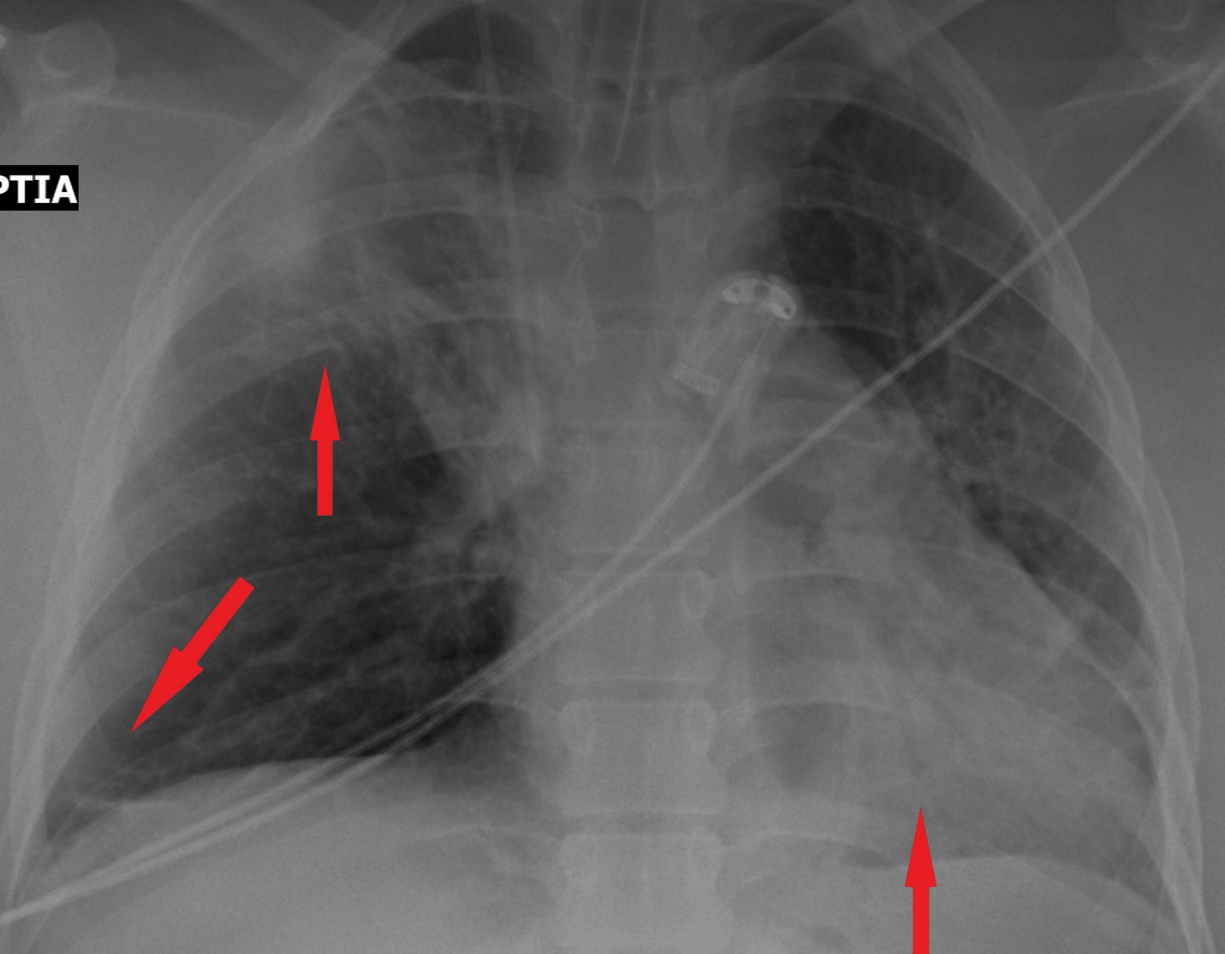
X-ray of chest with atelectasis of the upper right lobe and both lower lobes.
